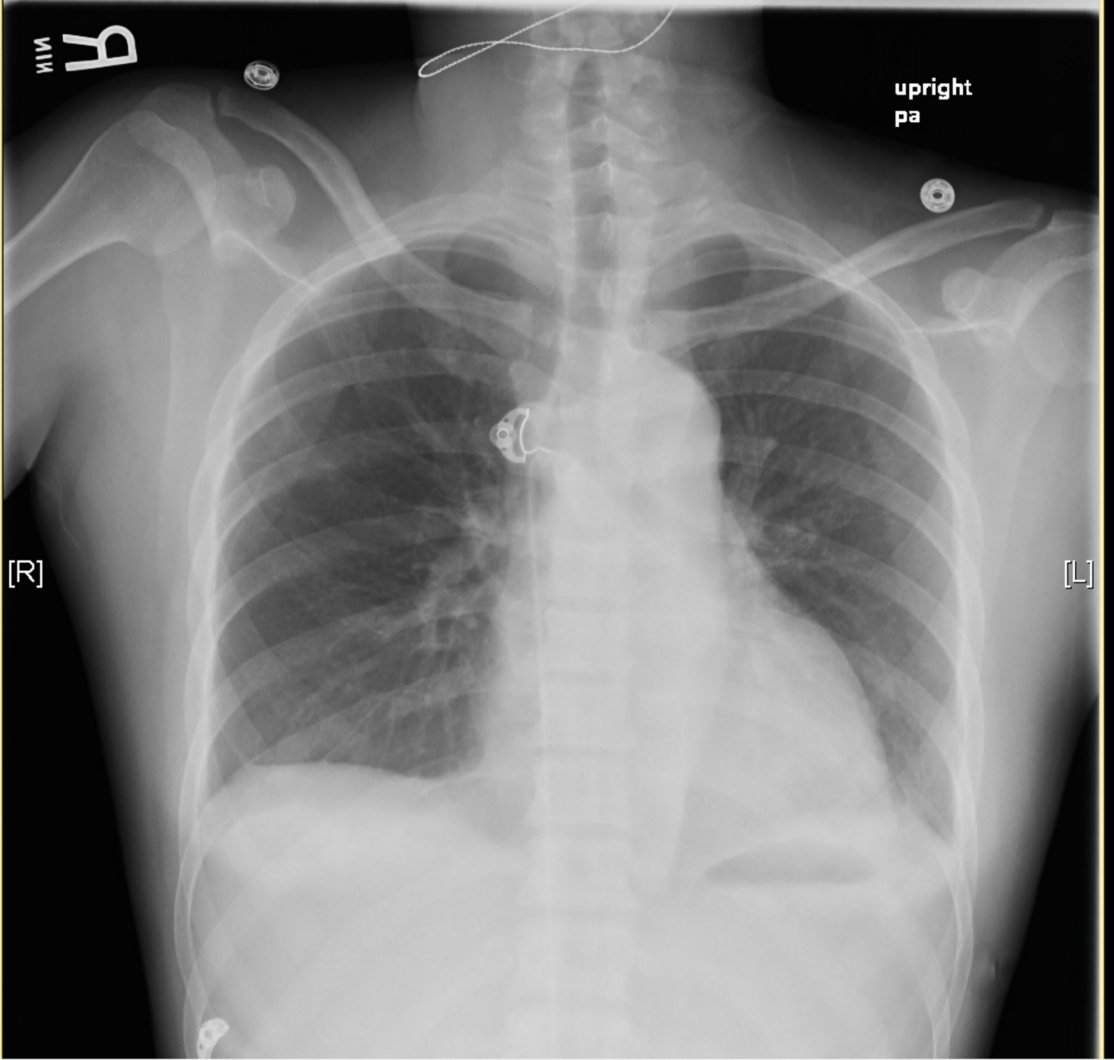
Chest X-ray upon presentation showing normal mediastinum
